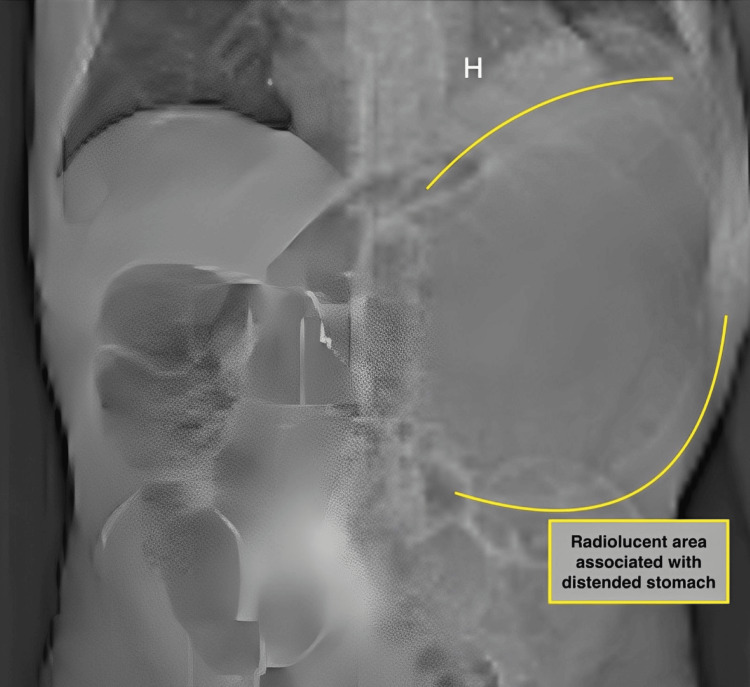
Abdominal X-ray showing a radiolucent area in the upper left quadrant corresponding to the stomach, which appears markedly distended
